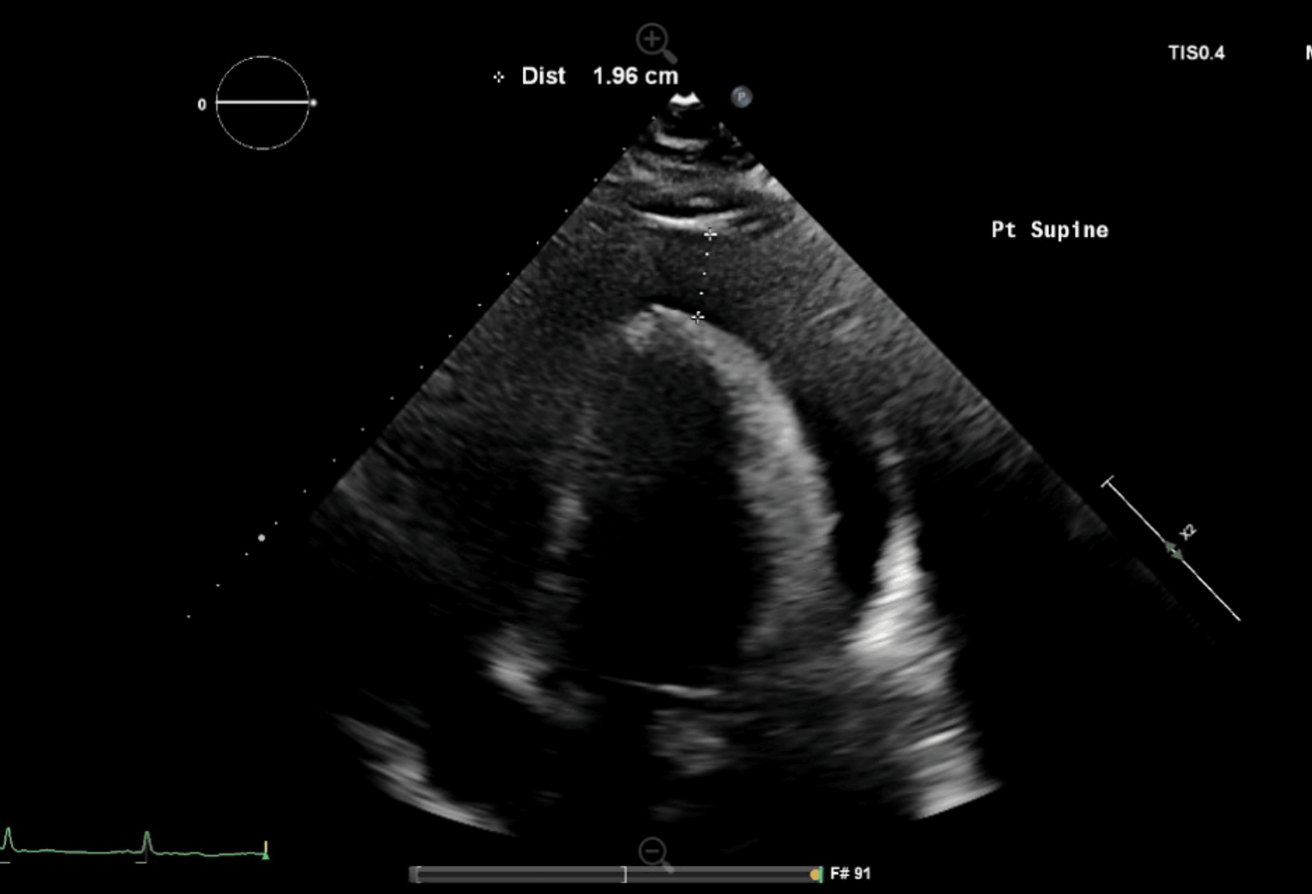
4-chamber view of a transthoracic echocardiogram demonstrating pericardial effusion with a maximum effusion width of 1.96 cm at the end of diastole.
